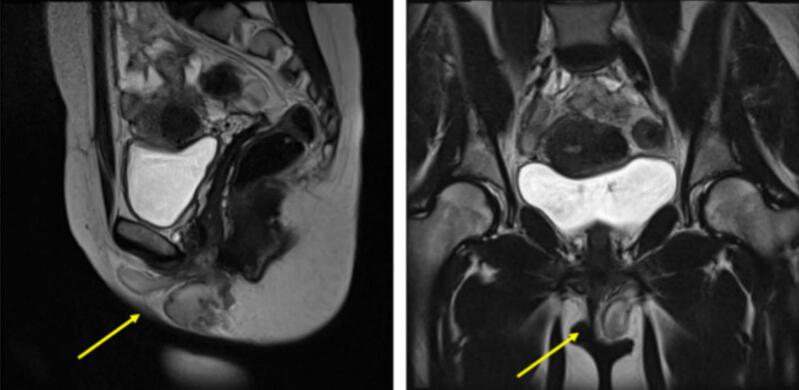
Sagittal and coronal T2 pelvic MRI: hypersignal myxoid mass of the left labia majora extending upwards into the ischio-rectal fossa, with no mass effect on adjacent organs.
